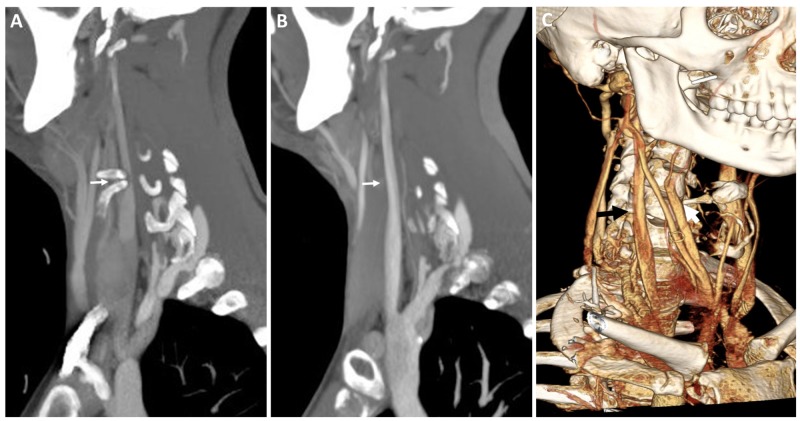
Three-dimensional reconstruction of postoperative vessel imaging. (A) Preoperative and (B) postoperative sagittal CTV images showing resolution of osseous compression (white arrows) by the hyoid bone. (C) Three-dimensional reconstruction of the postoperative CTV showing successful restoration of the IJV caliber (black arrow) and extent of hyoid resection (white arrow). CTV, computed tomography venogram; IJV, internal jugular vein
